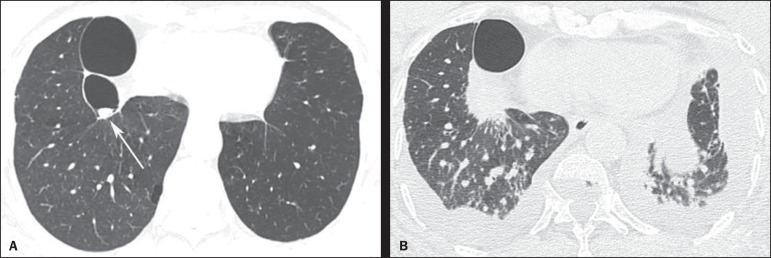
HRCT scan at the level of the lung bases (A) showing two bullae
